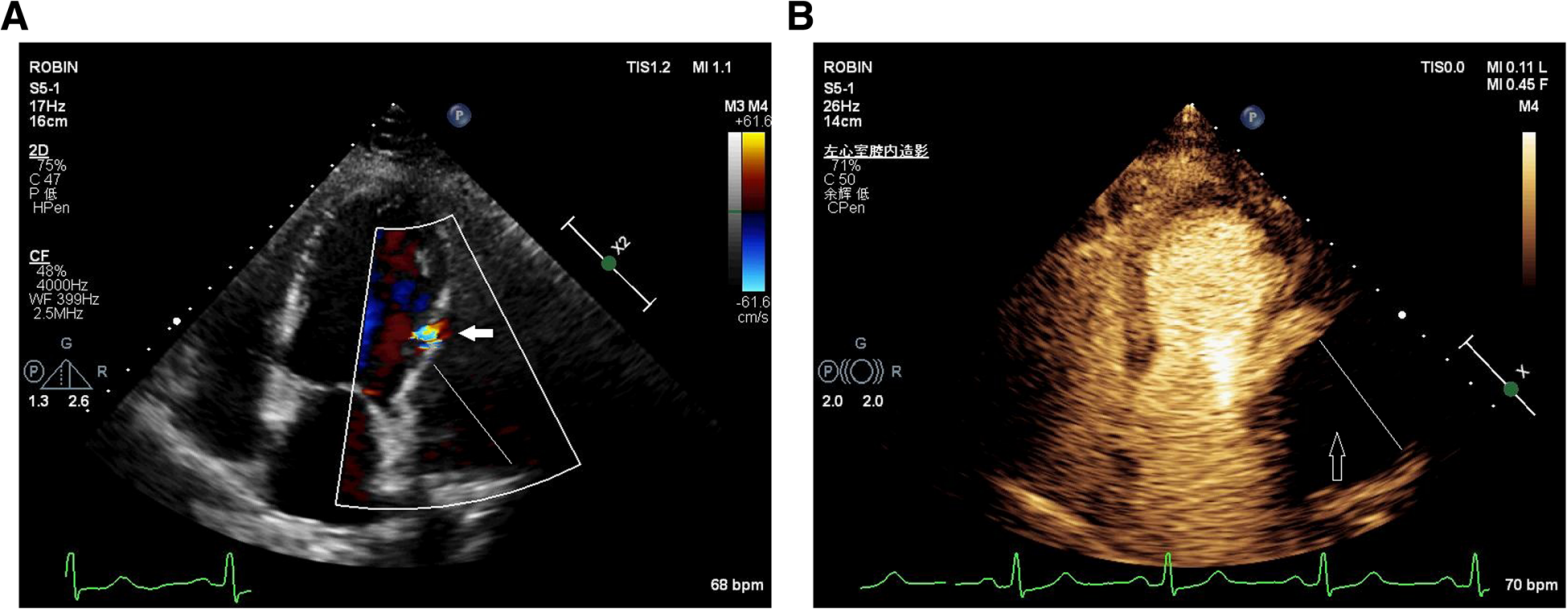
The picture a showed that the thrombus almost filled the aneurysm cavity. Partial blood flow imaging had been seen only nearby the defect of the left ventricular (indicated by the arrow). The picture b showed that myocardial contrast echocardiography clearly verified these findings. The black area (indicated by the arrow) without contrast agent filling suggested no blood flow into the pseudoaneurysm. It has been filled by thrombus completely
